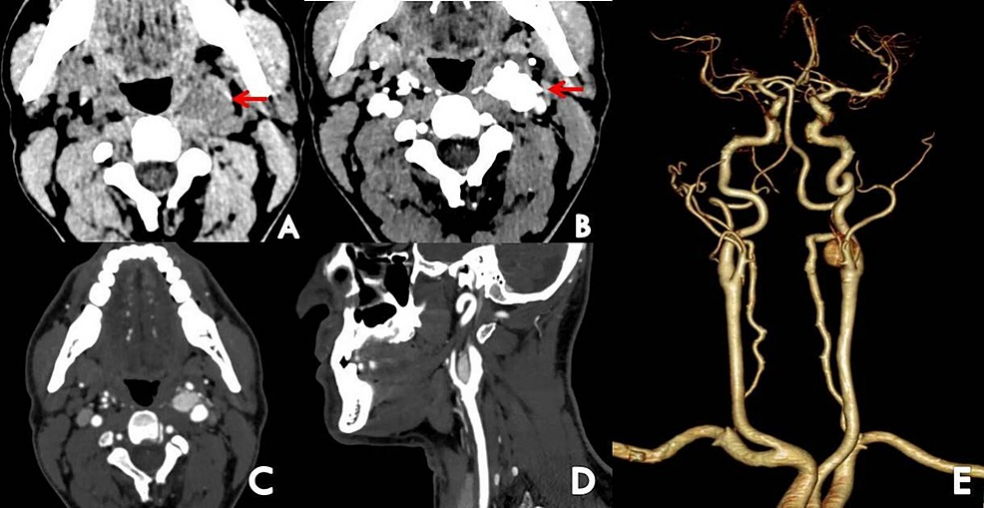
Plain (A) and contrast-enhanced (B) axial images of the neck and contrast-enhanced axial (C) and sagittal (D) CT reconstruction demonstrates a well-defined isodense mass lesion at the bifurcation of the left common carotid artery showing intense homogeneous post contrast enhancement (red arrows). 3D Coronal reformation from CT angiogram (E) showed the lesion causing splaying of the internal and external carotid arteries.
